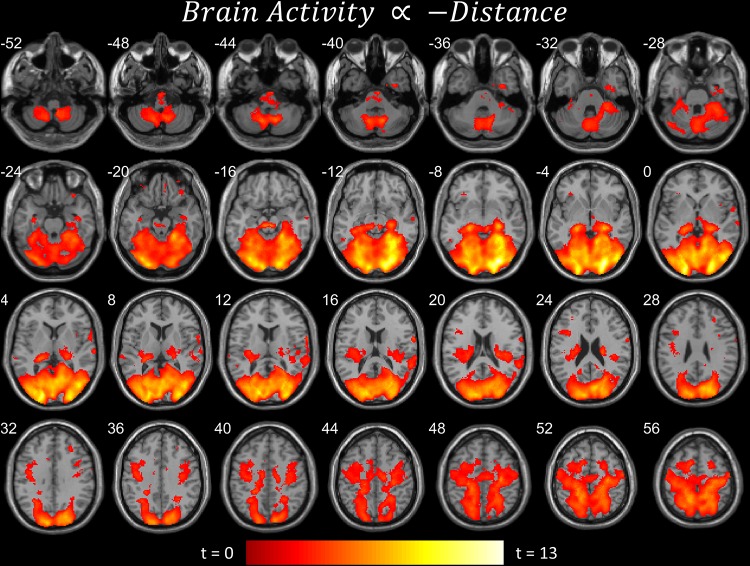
Brain areas that become more active when near the target. Significant parametric modulation (pFDR < 0.05 peak level corrected across entire brain) rendered on anatomical MRI slices using xjView toolbox (https://www.alivelearn.net/xjview). Note widespread, distributed activity, particularly in cerebellum and cortex.
